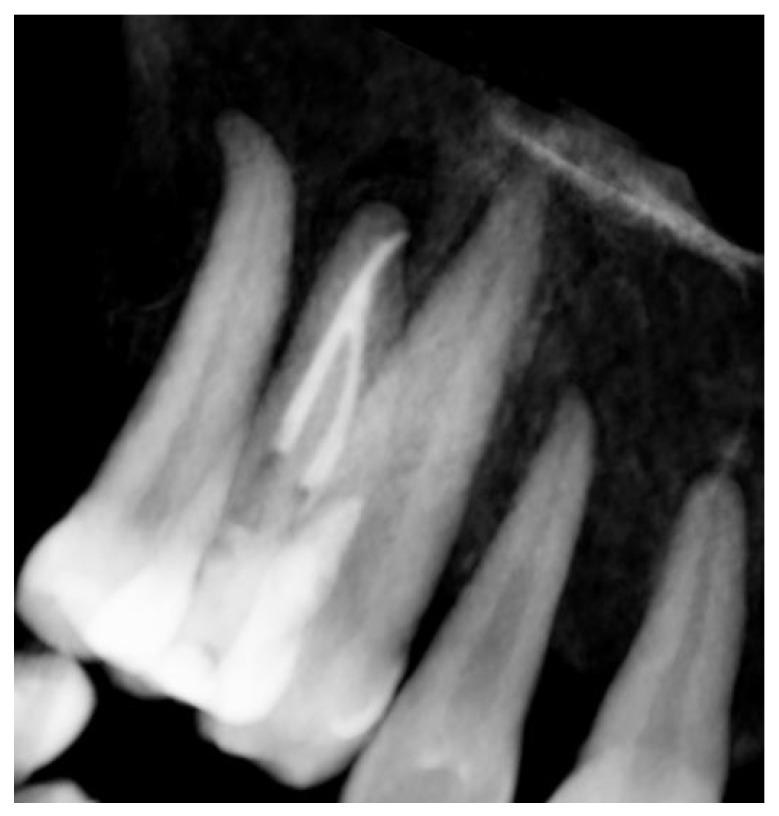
Rendering of CBCT images of tooth 1.4 after six months of healing.
